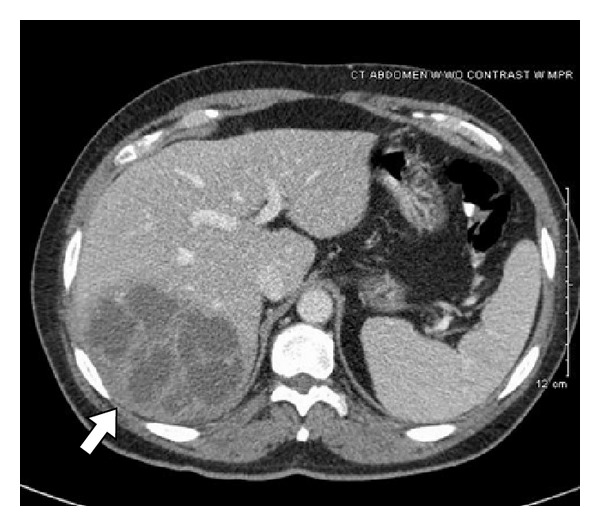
CT venous phase showing large hepatic abscess (arrow).
